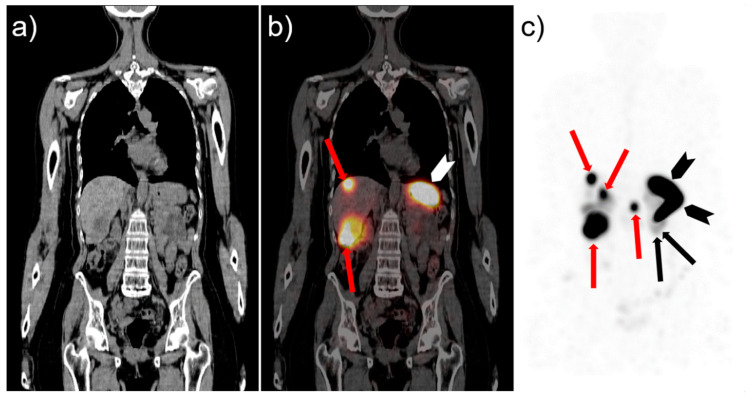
CT (a) and fused [^177^Lu]Lu-PP-F11N SPECT/CT (b) images in coronal orientation. Corresponding maximum intensity projection image of SPECT data (c). Images 72 h after infusion of 6.3 GBq (<100 µg peptide) in a female patient with hepatic MTC metastases demonstrates strong uptake of the radiopharmaceutical in hepatic metastases and stomach. Red arrows: Liver metastases; white and black arrowheads: stomach; black arrows: Kidney; courtesy of Dr. Christof Rottenburger.
